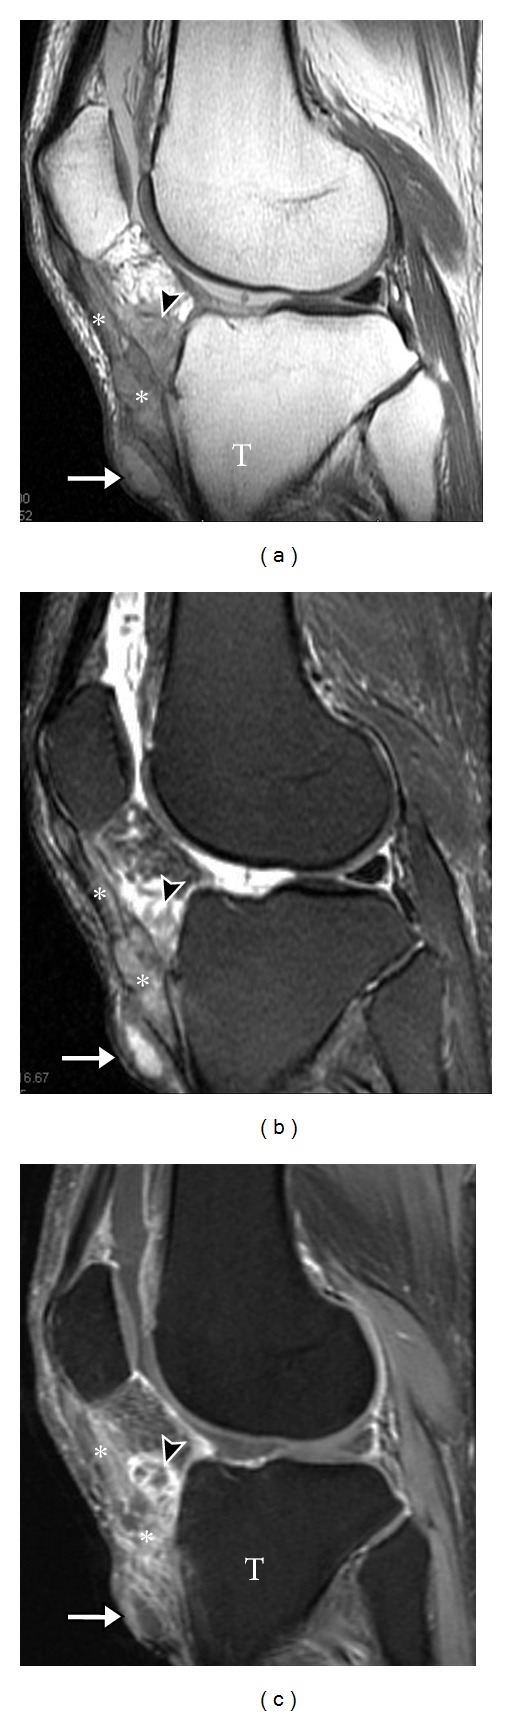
Intratendinous tophaceous gout on MRI. Sagittal T1 (a), sagittal T2 (b), and T1 postcontrast (c) MR images of the knee show abnormal, enhancing soft tissue gout deposit infiltrating the distal patellar tendon (asterisk) and extending across the facial planes to involve the adjacent Hoffa's fat pad (arrow head) and pretibial subcutaneous tissue (long arrow). T: tibia.
